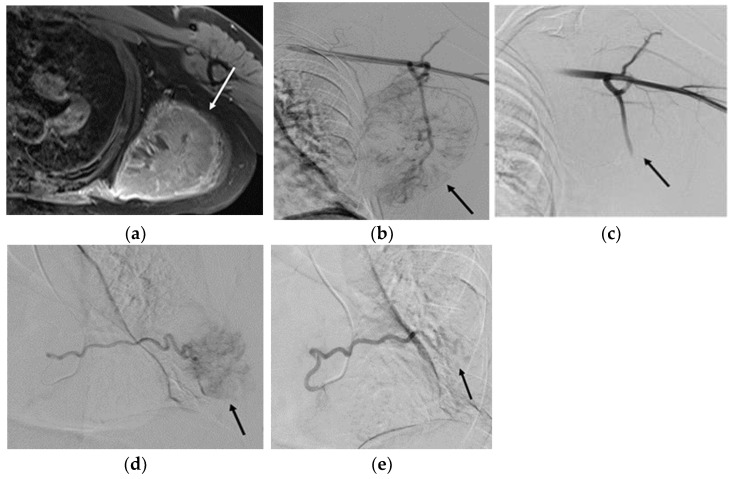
A 47-year-old male with a history of metastatic renal cell carcinoma with a left scapula soft tissue metastasis: (a) MRI of the left shoulder with contrast demonstrates a solid enhancing mass in the left scapula (arrow) measuring 8.8 × 9.2 × 9.3 cm with extension into the adjacent teres major and teres minor, subscapularis, and infraspinatus muscles with associated erosion of the inferior scapula. (b) Selective angiography of the left axillary artery demonstrates arterial supply via the lateral thoracic artery to the tumor (approximately 3/4 of the tumor volume). Gelfoam^®^ and particle embolization of the lateral thoracic artery were performed. (c) Post-embolization angiography of the lateral thoracic artery demonstrates satisfactory stasis and decreased tumor vascularity. (d) Selective angiography of the left seventh intercostal artery demonstrates arterial supply to the tumor (approximately 1/4 of the tumor volume). (e) Post-embolization angiography shows decreased tumor vascularity. MRI, magnetic resonance imaging.
